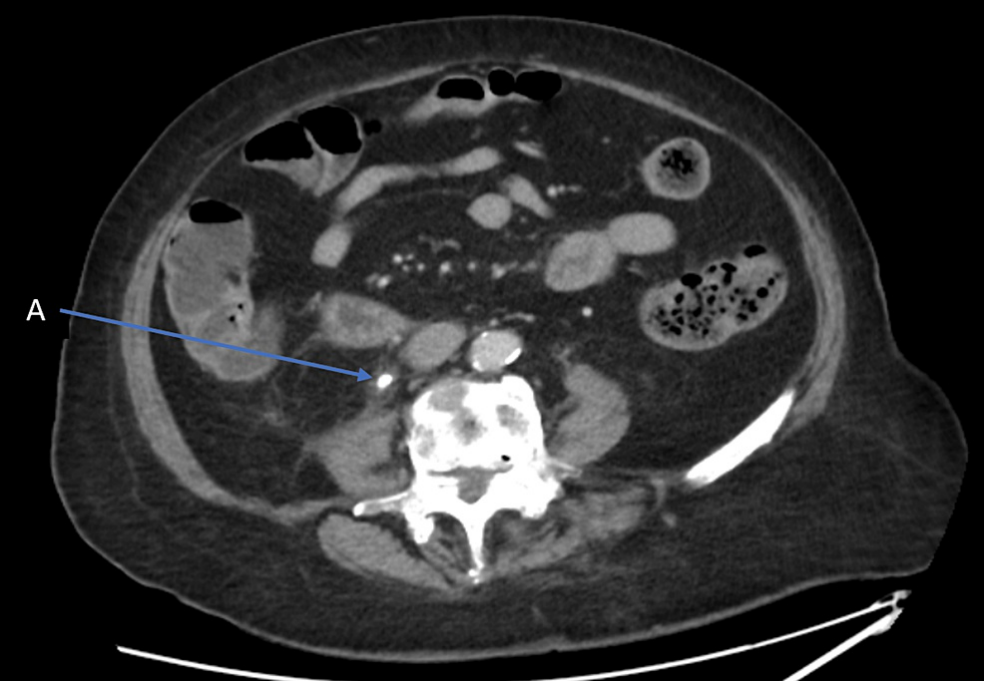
Axial view of contrasted computed tomography of the abdomen and pelvis showing impacted right-sided pelvic-ureteric junction stone (A).
